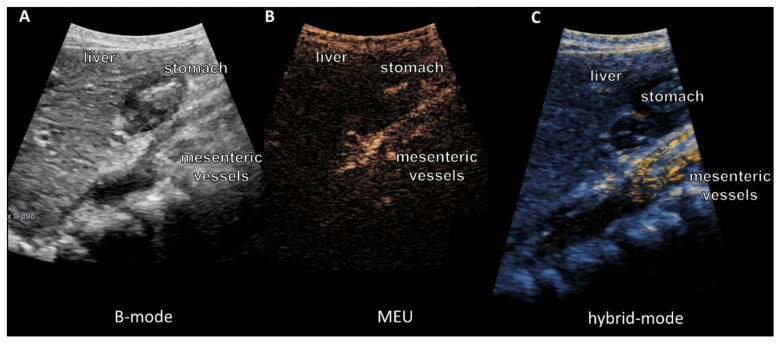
High resolution Ultrasound, multi-frequency C1-6 MHz probe, direct comparison of B-mode (A), microbubble enhanced ultrasound (MEU) (B), and hybrid mode (C) 90 minutes after liposomal vitamin C uptake. (A) B-mode after application of the microbubbles with vitamin C (B): enhanced signal in the subtraction image with low MI ≤ 0.16, high signal intraluminal in the stomach-duodenum, mesenteric vessels, and liver corresponding to the enhancement of the MEU. (C) Visualization of the microbubbles with the hybrid subtraction mode. Clear enhancement of the bubbles in the intraluminal vessels, stomach/duodenum, and liver.
